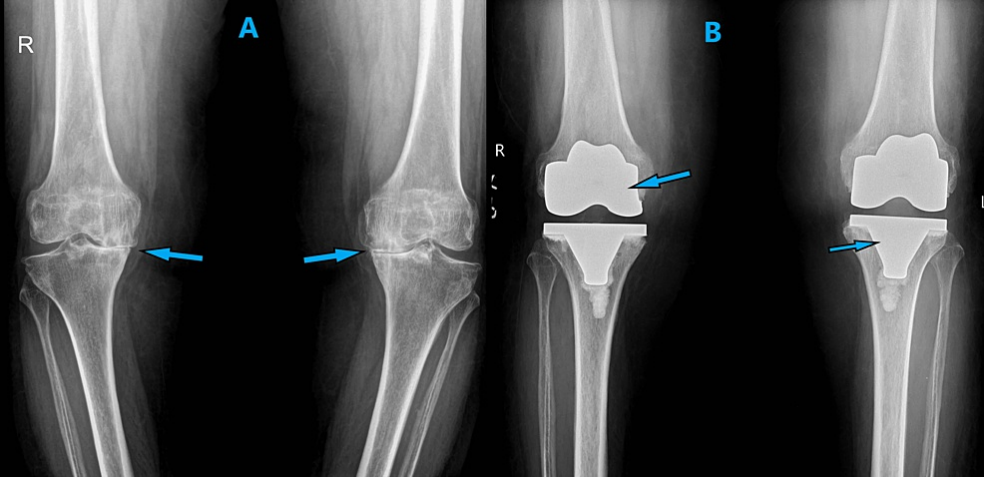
A) Preoperative X-ray of both knee joints showing features of arthritis and varus deformity. B) Postoperative X-ray showing good alignment and positioning of total knee arthroplasty implants
